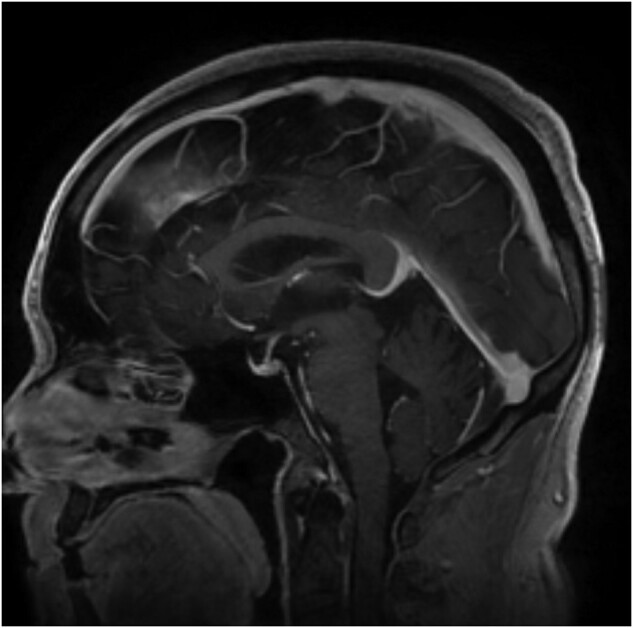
MRI following withdrawal of pembrolizumab and initiation of steroid therapy.
